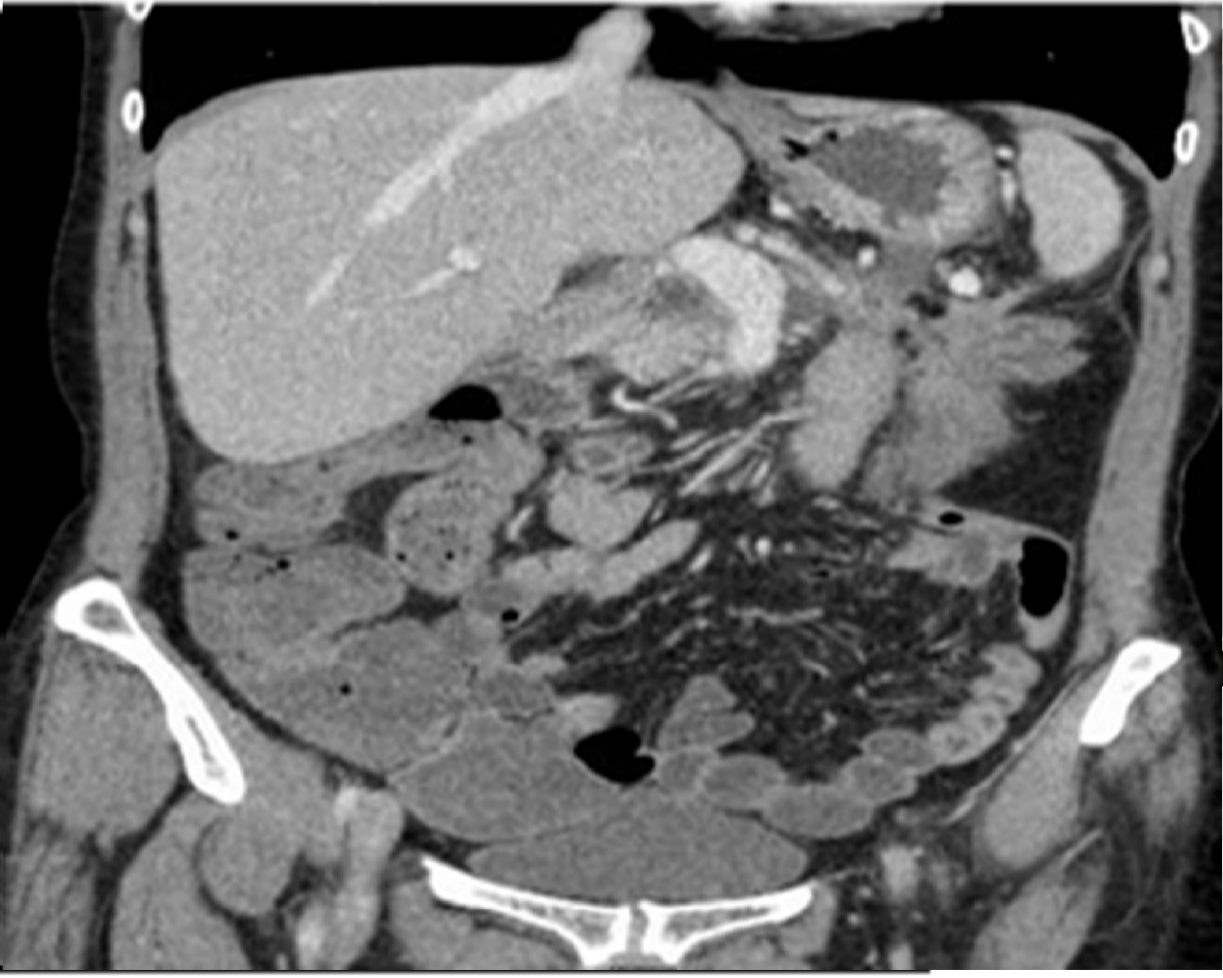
CT Abdomen and Pelvis with IV Contrast Coronal CT images of the abdomen and pelvis demonstrating fluid-filled small bowel loops with mild-moderate wall thickening.
